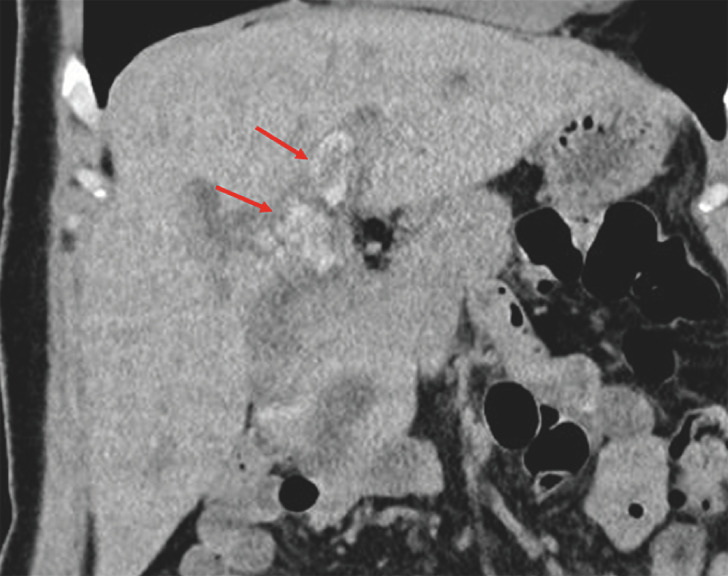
Computed tomography revealed large stones (red arrows) in the intrahepatic bile duct.
